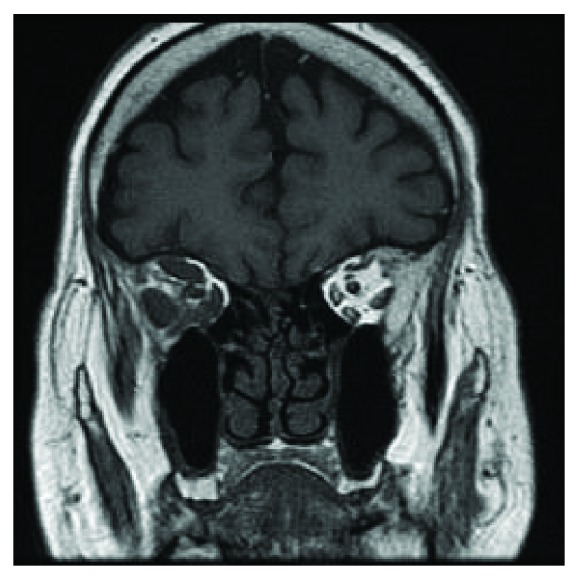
Coronal T1 MRI demonstrating enlargement of all of the extraocular muscles of the right orbit.
